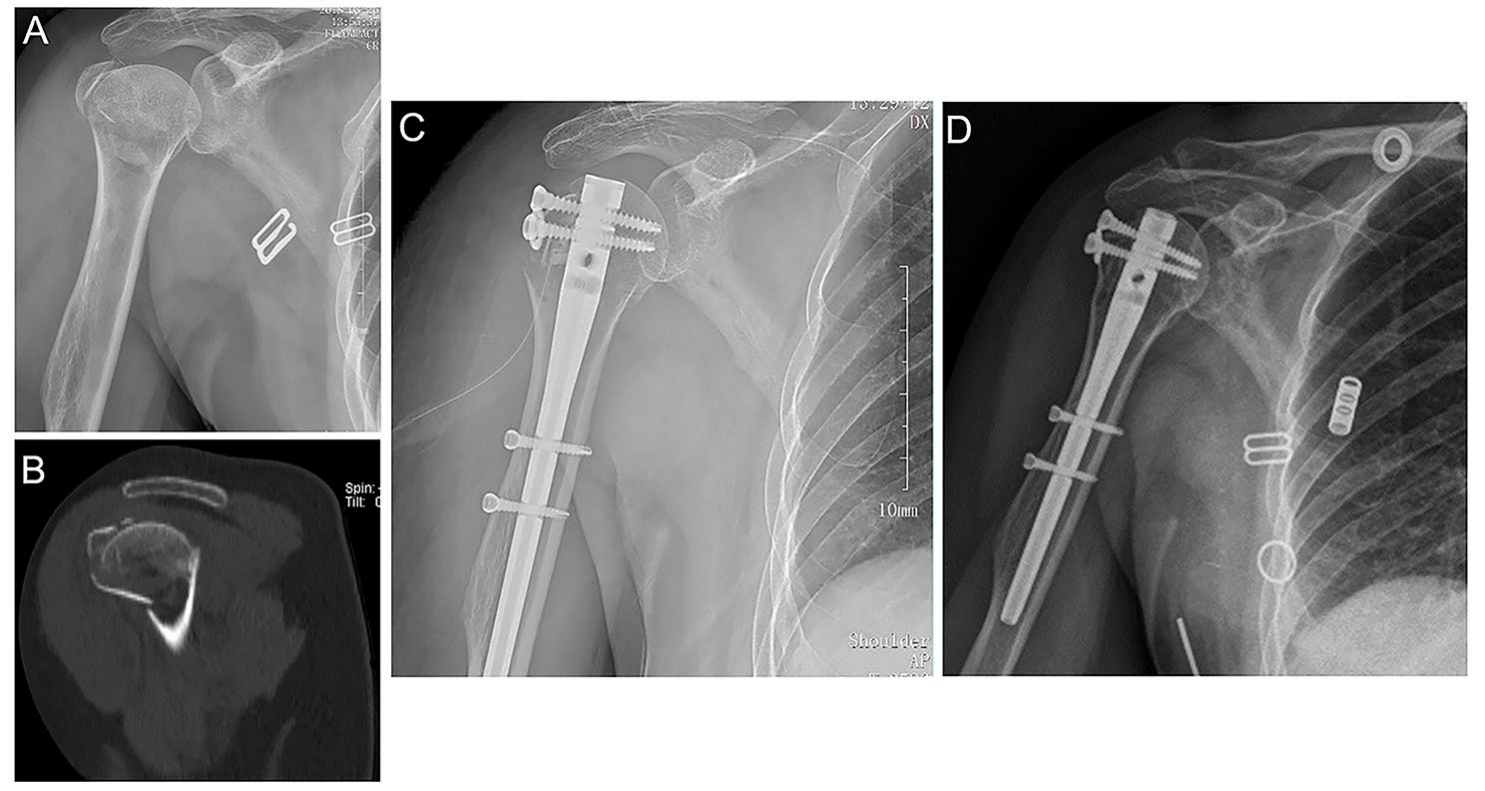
An example case of a right proximal humerus fracture in a 54-year-old woman treated with intramedullary nail fixation. (A) X-ray image showing the fractures in the anatomical neck of the right humerus and the greater tuberosity, as well as valgus cottage and greater tuberosity displacement; (B) CT scan of the fracture and displacement; (C) Postoperative anteroposterior X-ray image showing good reduction of the anatomical neck and greater tuberosity fracture. The proximal and distal interlocking screws satisfactorily positioned; (D) Anteroposterior X-ray image of the right humerus anatomical neck and greater tuberosity showed good healing, no loosening of internal fixation, and no displacement of fracture at one year postoperatively. CT, computed tomography
